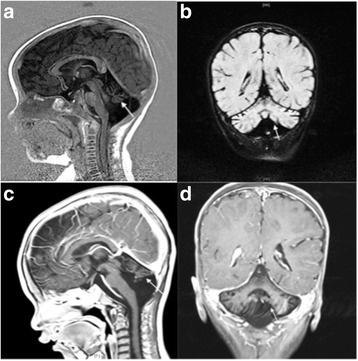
a At our patient’s first visit (8-months old), cranial magnetic resonance imaging showed severe atrophy of cerebellar hemispheres; coronal position (*arrow*). b Severe hypoplasia of cerebellar vermis was noted at sagittal position on cranial magnetic resonance imaging (*arrow*). c When he was 3-years old, the atrophy of cerebellar hemispheres still existed (*arrow*) and the volume of cerebellar hemispheres was evidently less than the average level of his peers. d The absence of cerebellar vermis was noted obviously (*arrow*)
